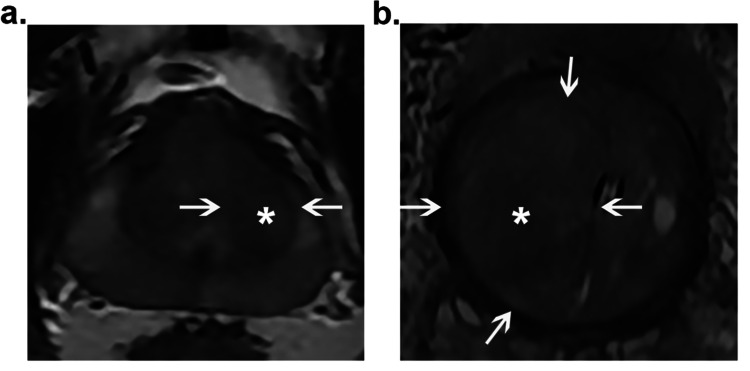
The confirmation process of tumor location. (a) A 60-year-old man with mild urinary symptoms. This patient underwent MR imaging and was diagnosed with cancer after prostatectomy. (b) A 67-year-old man with severe urinary symptoms. This patient underwent an MRI-guided biopsy and was diagnosed with benign prostatic hyperplasia.
